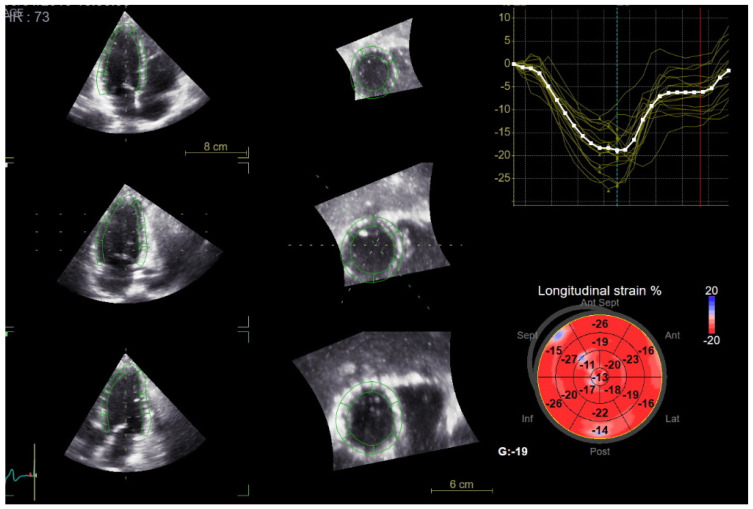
Representative example of four-dimensional speckle-tracking echocardiographic analysis of the left ventricle, demonstrating three-dimensional endocardial tracking and quantitative assessment of global strain parameters (GLS). The thin colored solid curves represent segmental longitudinal strain, whereas the thick white dashed curve represents the global longitudinal strain curve.
